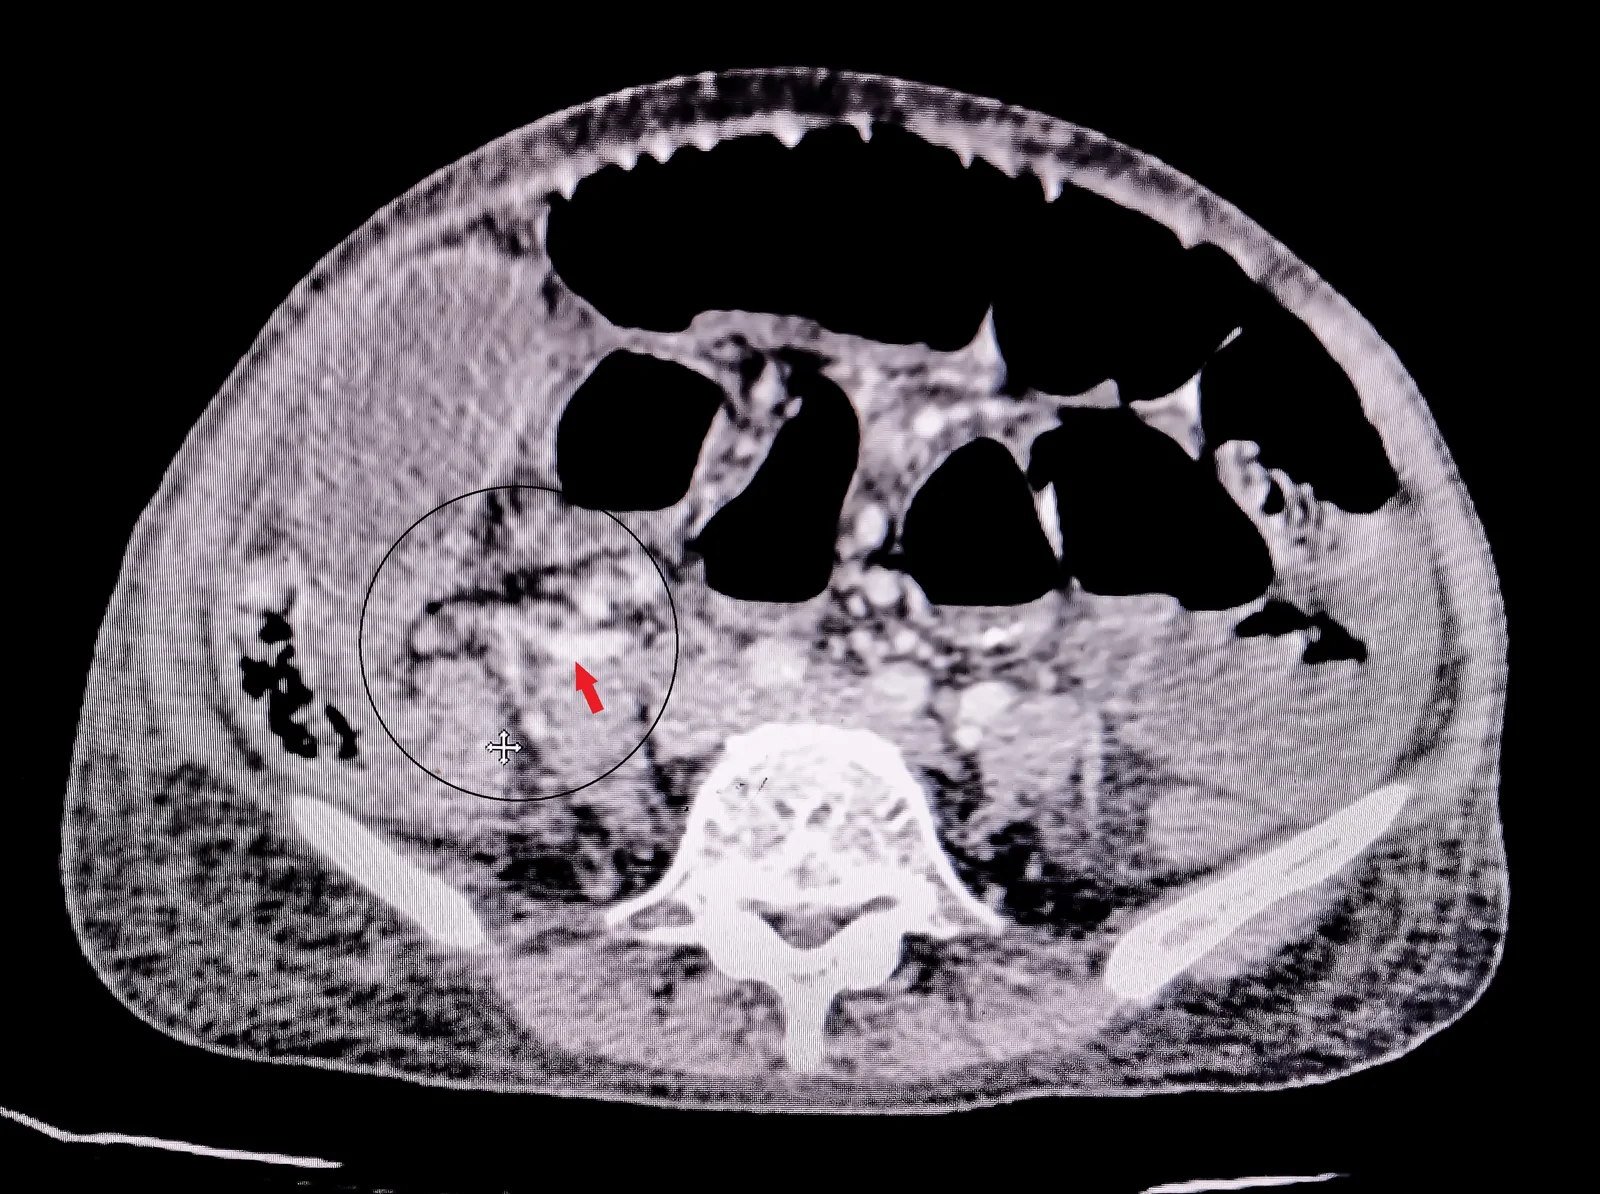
Contrast-enhanced computed tomography (CECT) whole abdomen showing twisted and conglomerated distal ileum and its twisted vasculature Distal ileum and its twisted vasculature (encircled); twisted vessels in the mesentery (marked with red arrow).
